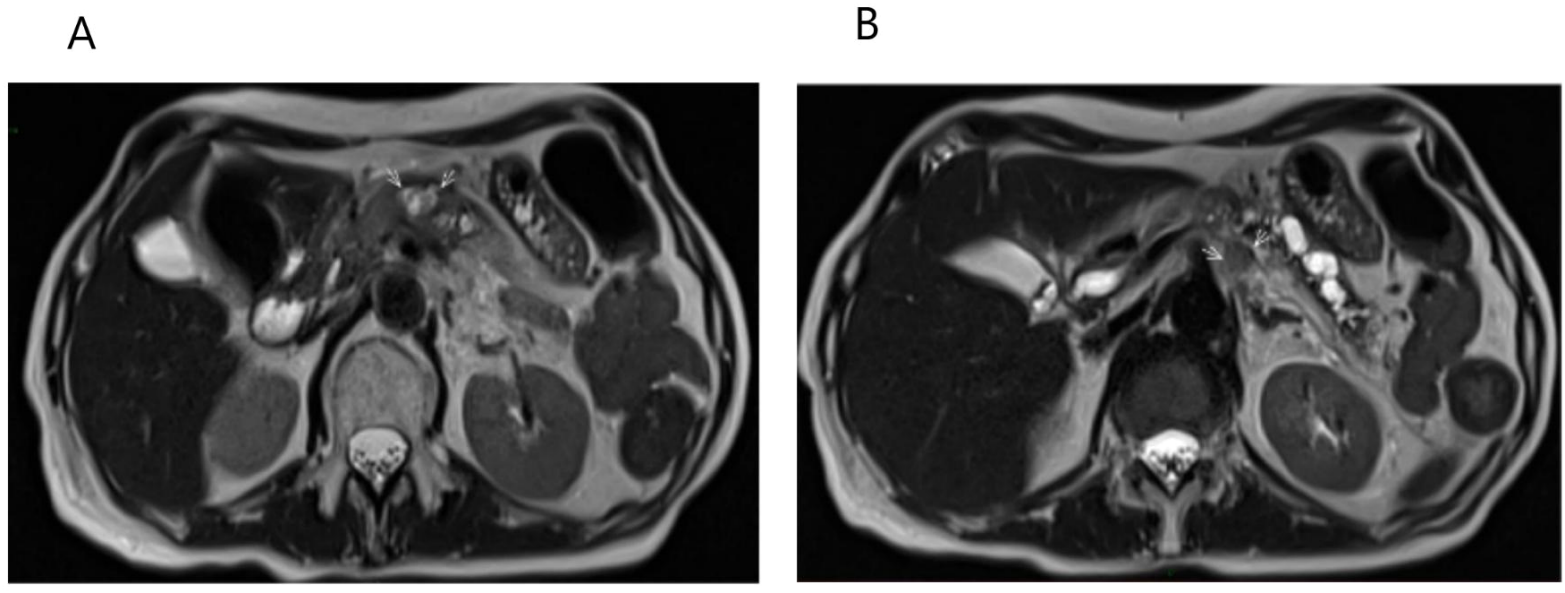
Radiologic evaluation of the pancreatic lesion and regional lymph node after systemic chemotherapy. (A) Axial T2-weighted MRI reveals an oval, heterogeneous area measuring 1.8 x 2.4 cm, containing small necrotic foci and restricted water diffusion, indicating residual active tumor tissue. (B) Axial T2-weighted MRI shows a slightly enlarged oval lymph node near the celiac trunk, measuring 1.3 x 1.1 cm, also with restricted water diffusion.
